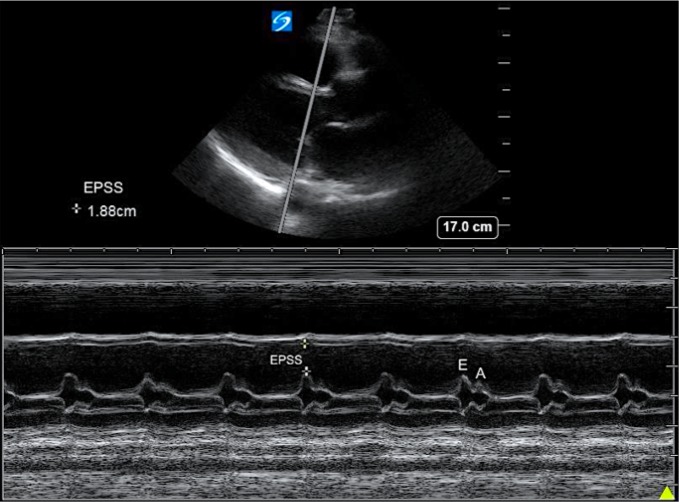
An M-mode ultrasound scan through the distal- Most aspect of the anterior leaflet of the mitral valve to trace the movement of the anterior MV leaflet through the cardiac cycle. The E and A points of each diastole are visible as asymmetric humps during each diastole.
